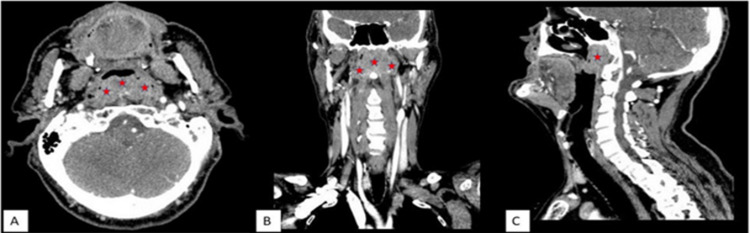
Contrast-enhanced CT images From left to right, axial (A), coronal (B), and sagittal (C) plane images The mass largely obliterates the nasopharyngeal air column, with lobulated contours, heterogeneous contrast (containing non-enhanced areas that may belong to cystic-necrotic areas), causing bilateral mastoid effusion by filling the posterior and both lateral walls of the nasopharynx (bilateral lateral pharyngeal recesses = Rosenmüller fossa) more prominently on the left. The mass had 22x52x36 mm (anterior-posterior x transverse x craniocaudal) dimensions (red asterisks). There were no bony erosion in skull base or enlarged cervical lymph node in the neck.
